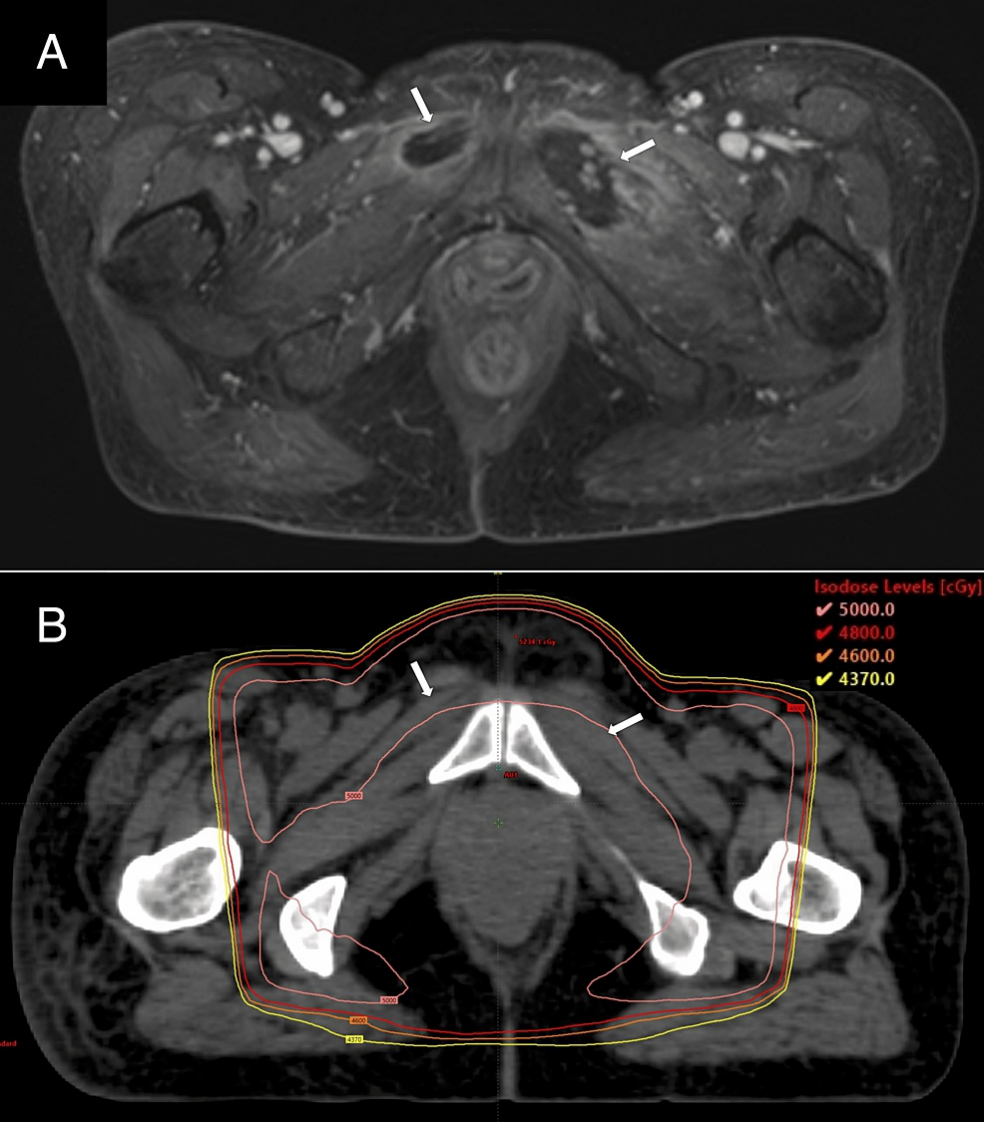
Contrast-enhanced MRI axial image (A) and planning CT axial image showing the dose distribution (B) (A) Contrast-enhanced MRI showed poor contrast mass in the left obturator externus muscle and right pectineus muscle (white arrows). (B) The location of mass was equivalent to 50 Gy in isodose level with a pink line. MRI: magnetic resonance imaging; CT: computed tomography
